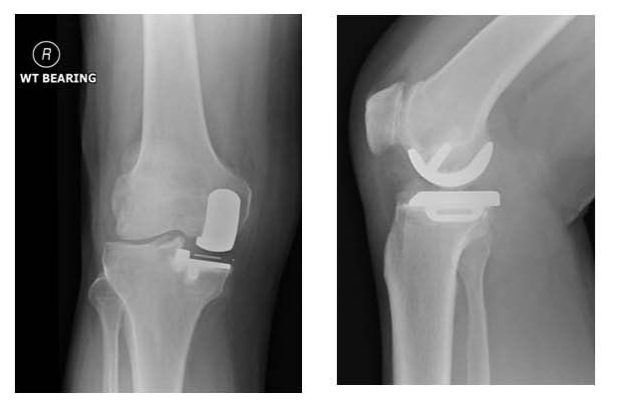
Pre-operative anteroposterior and lateral radiographs of the patient showing medial unicompartmental prosthesis in-situ.
